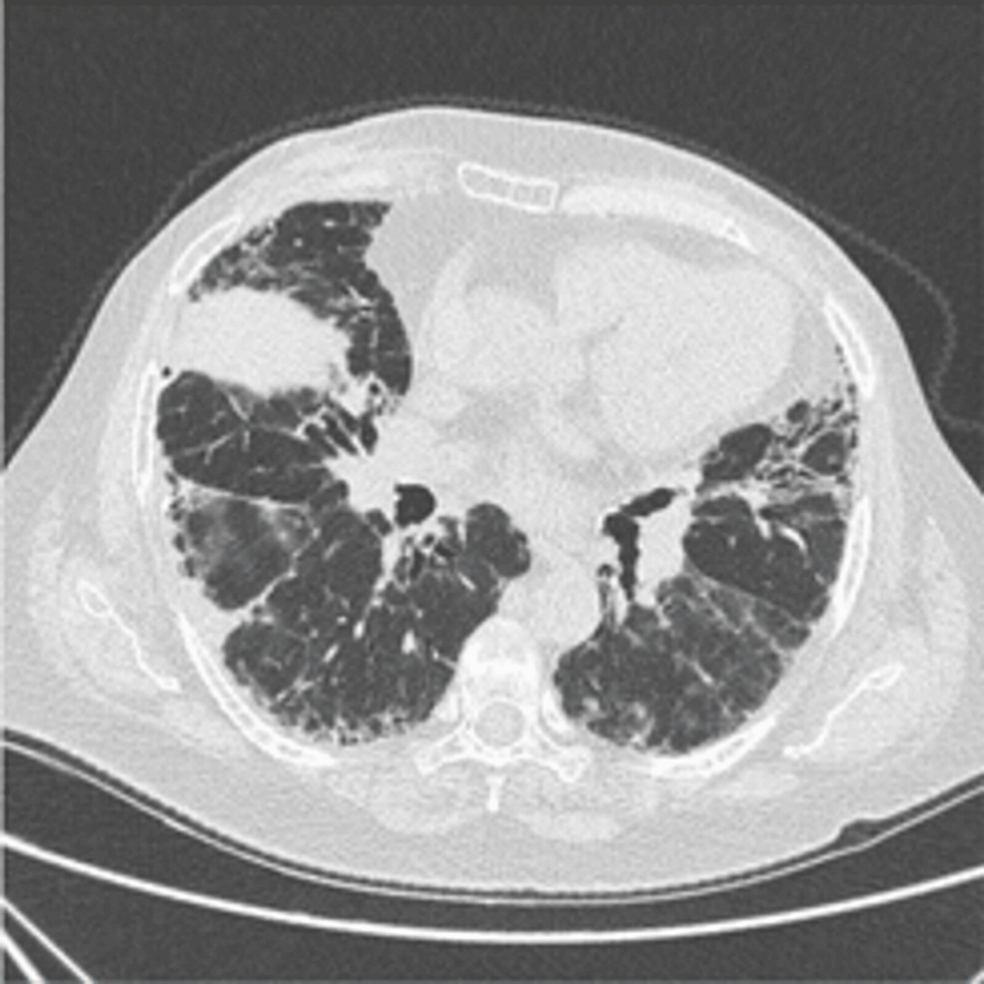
High-resolution computed tomography (CT) scan of the chest showing basilar predominant coarse interlobular septal thickening with peripheral bronchiolectasis. Early subpleural is honeycombing within both lung bases.
